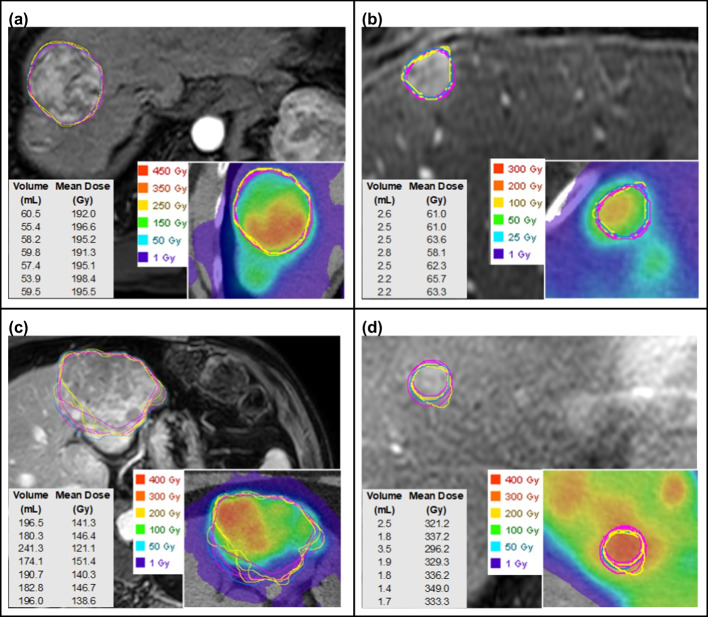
Radiologist-defined tumor contours on MRI corresponding to the four example lesions depicted in Fig. 1. Inserts show contours transferred to co-registered Y-90 PET/CT-based dose maps. Mean dose values are indicated before PVC. a Large, well defined (code 46.1). b Small, well defined (39.3). c Large, poorly defined (49.1). d Small, poorly defined (55.4). Contours drawn by the same radiologist are indicated in the same color (Pink = radiologist A, Yellow = radiologist B, Blue = radiologist C)
